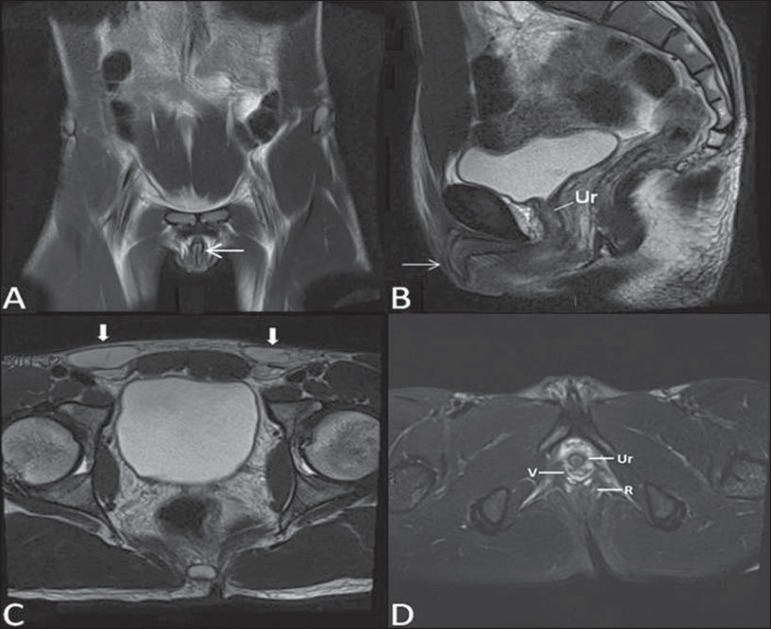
Partial androgen insensitivity syndrome. Multiplanar MRI T2- weighted sequences
